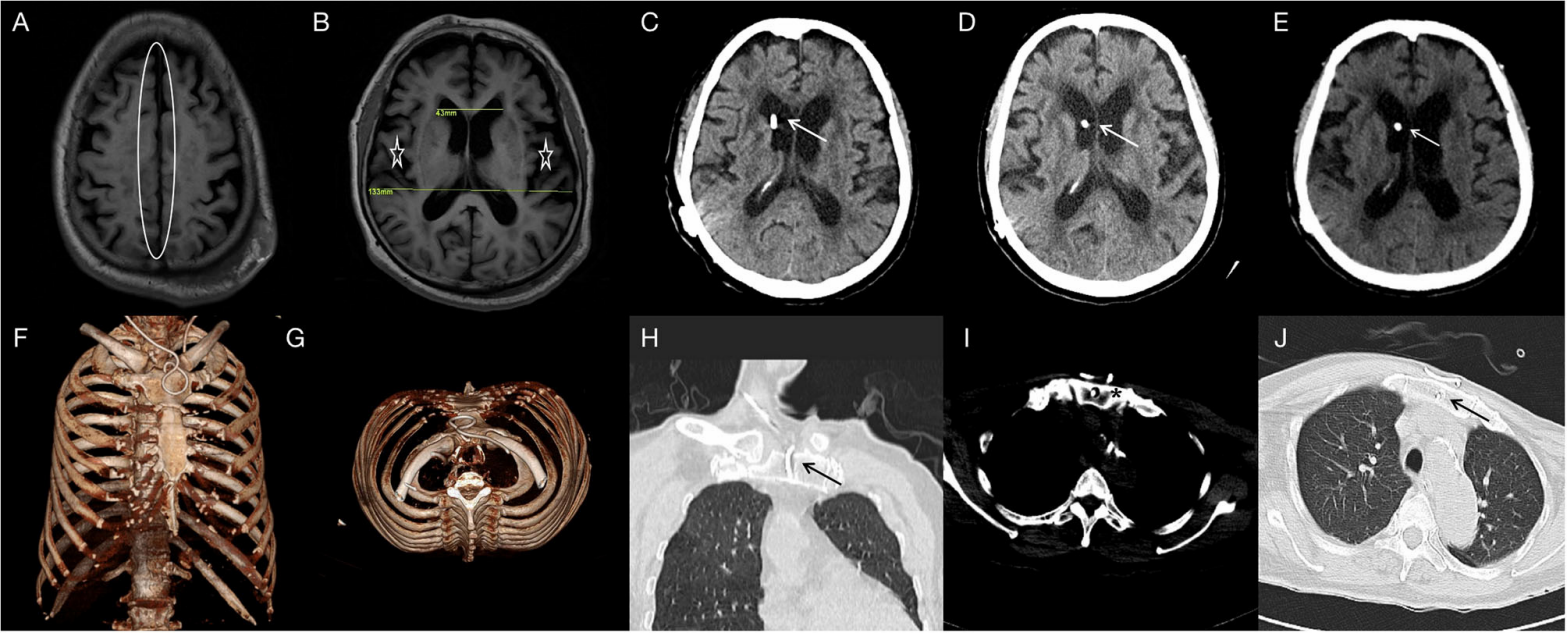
T1FLAIR (A,B) brain MRI showing the narrowed sulcal spaces at the vertex (oval) and the enlarged ventricles of the brain and lateral cracks(asterisk) (DESH); Evans Index was 0.32. Two-month CT-(C), 6-month CT-(D) and 16-month CT-(E) brain scan showing the catheter in position and the hydrocephalus is relieved. One-week three-dimensional reconstructed CT- (F,G) scan of the thorax showing the distal catheter *in situ*. Two-month CT- (H,I) and 16-month CT-(J) thorax scan showing the sternum is intact and the distal catheter *in situ*.
